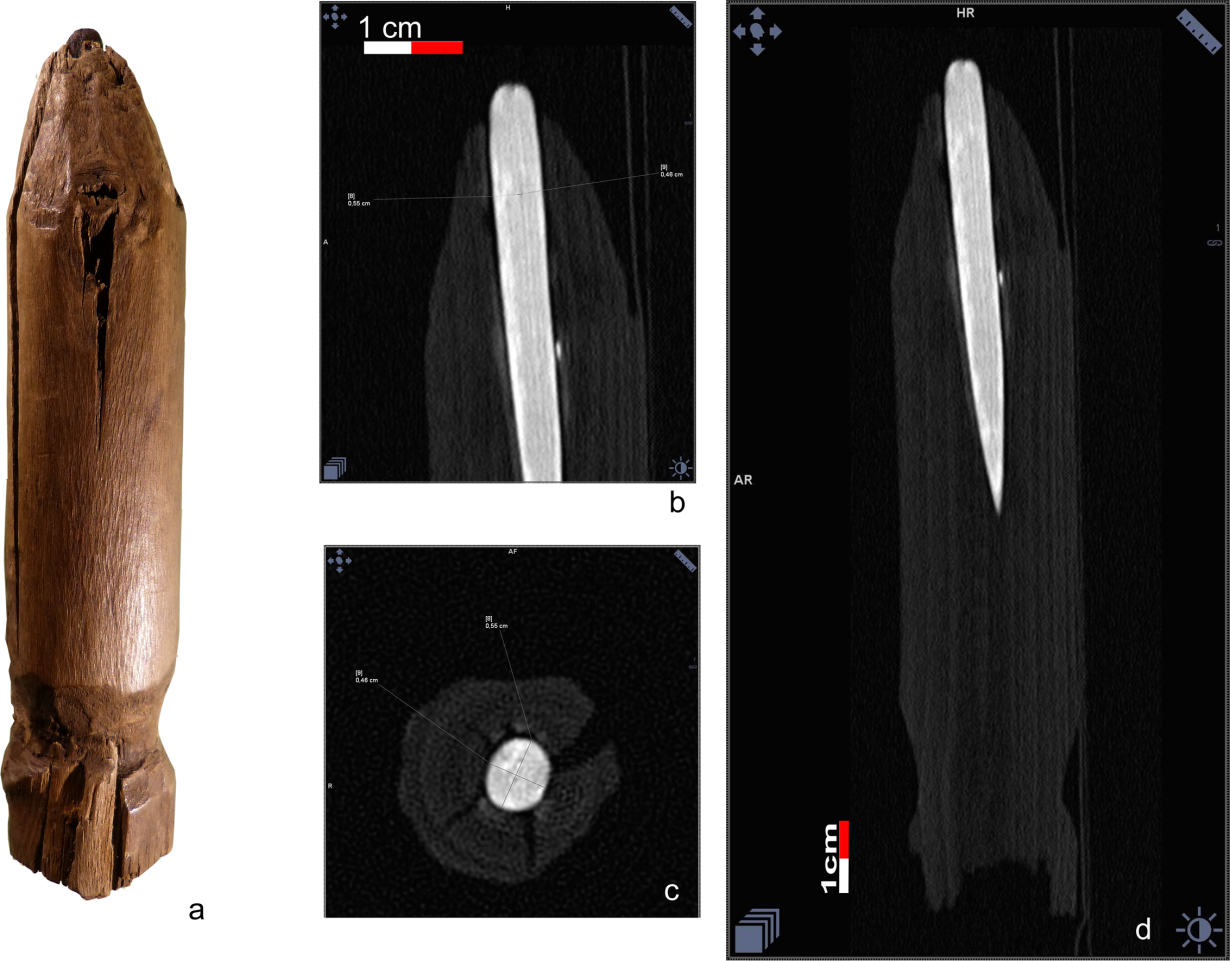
Retoucher. a) the wooden handle and antler tip, b-c) detail of the radiological structure of the antler spike, displaying the cortical and the cancellous parts, d) CT image depicting its radiological structure.
